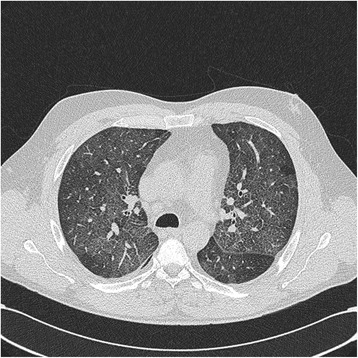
HRCT of thorax on the second day of hospitalization showed extensive bilateral areas of ground-glass opacities predominantly in the lower regions of the upper lobes, the right middle lobe and the apical regions of the lower lobes, compatible with severe chemical pneumonitis
